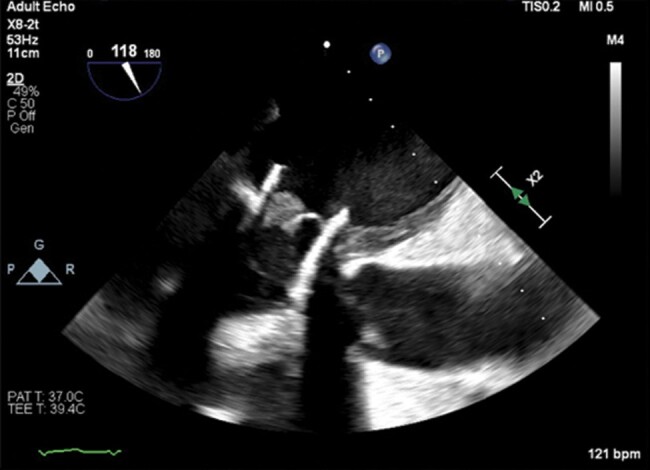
Transoesophageal echocardiogram reveals a dilated left atrium with an isoechoic mass straddling the leaflets of the new biological prosthetic valve [94 × 129 mm (×DPI)].
